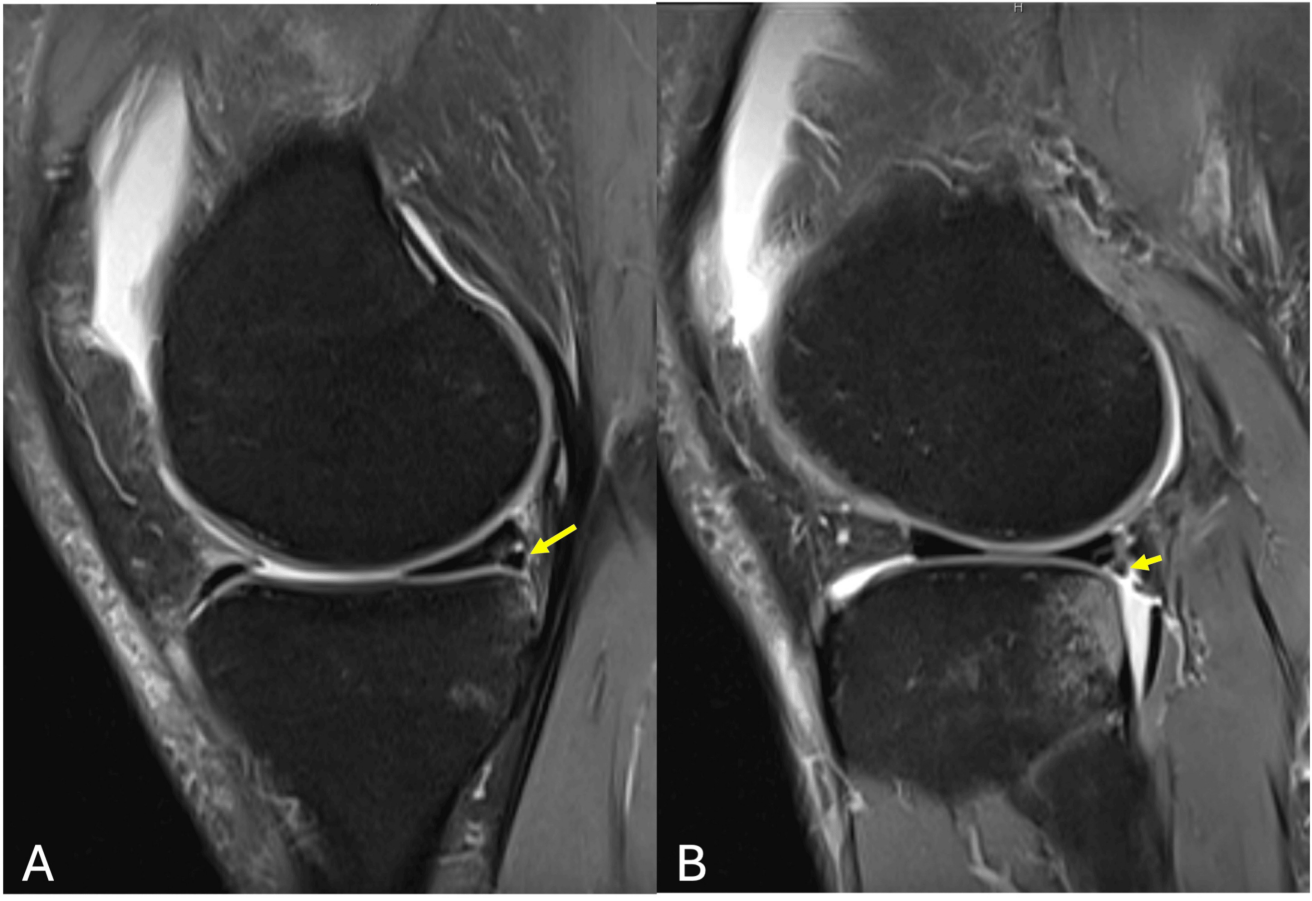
Sagittal PDFS MRI showing (A) small flap tear of posterior horn of medial meniscus and (B) small flap tear of posterior horn of lateral meniscus (yellow arrows) PDFS : proton density fat-saturated
